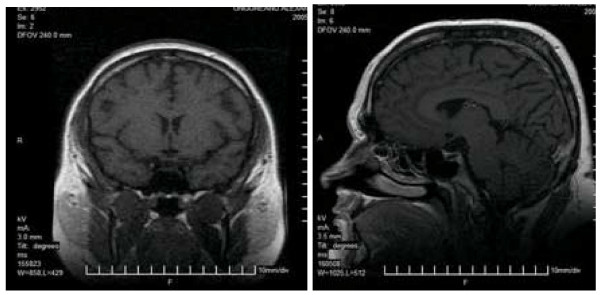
Patient 2. Normal MRI study of the diencephalic and pituitary region.
